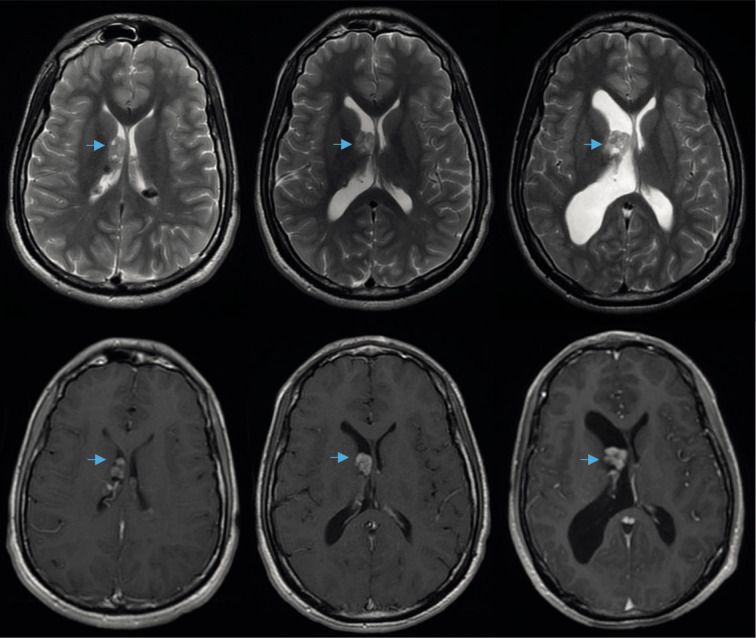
Brain MRI study from successive biannual controls. The upper images are axial T2 cuts, and the lower corresponding images are in T1 sequence with gadolinium. There is an identified enhancement with progressive increase in the size of the subependymal nodule (blue arrows) and the progressive enlargement of the right lateral ventricle.
